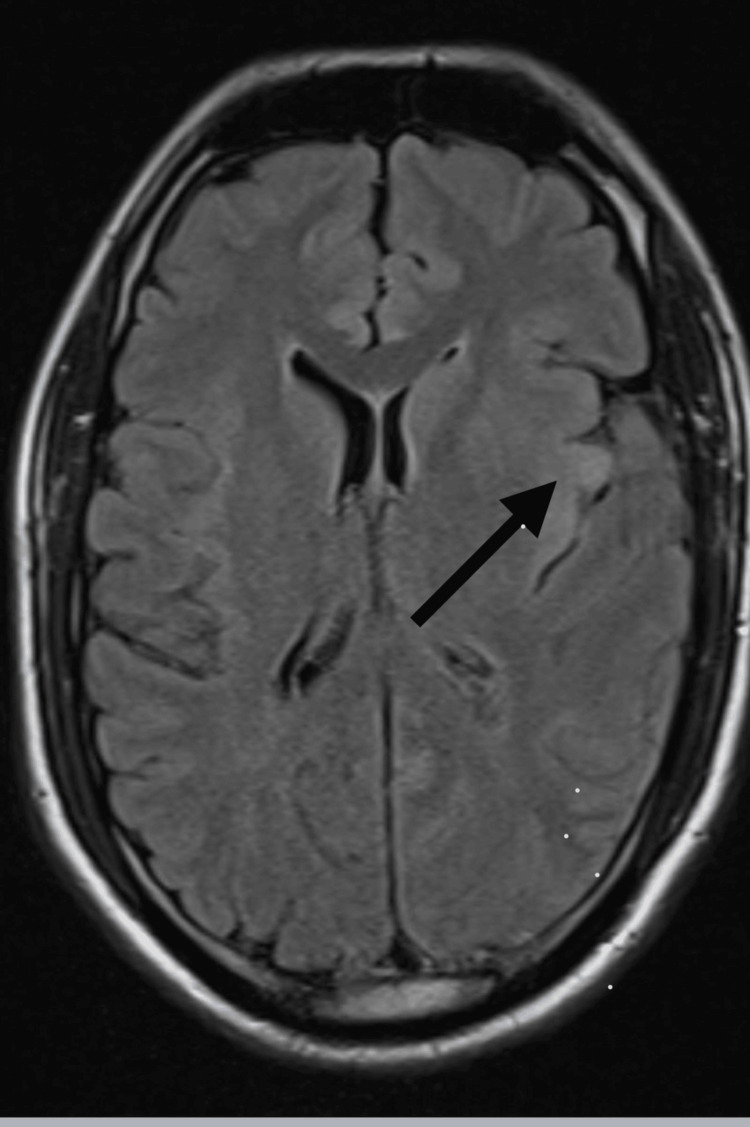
MRI brain axial FLAIR image taken on January 24, 2024, showing left insular hyperintensity with effacement of the left frontal horn (black arrow), and subtle involvement of the lentiform nucleus, frontal operculum, and cingulate gyrus. FLAIR: Fluid-attenuated inversion recovery.
